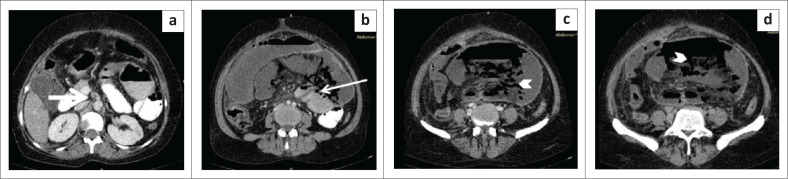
Superior mesenteric artery (SMA) thrombosis with acute mesenteric ischaemia. Axial contrast enhanced CT images (a–d) reveal thrombus in the superior mesenteric artery (thick white arrow in a) at the level of its origin from aorta. The large and small bowel loops appear dilated and show paper-thin walls. Few air foci are seen within wall bowel loops suggestive of pneumatosis intestinalis (thin white arrow in b). Air is also noticed along the mesenteric vessels (arrowheads in c and d).
